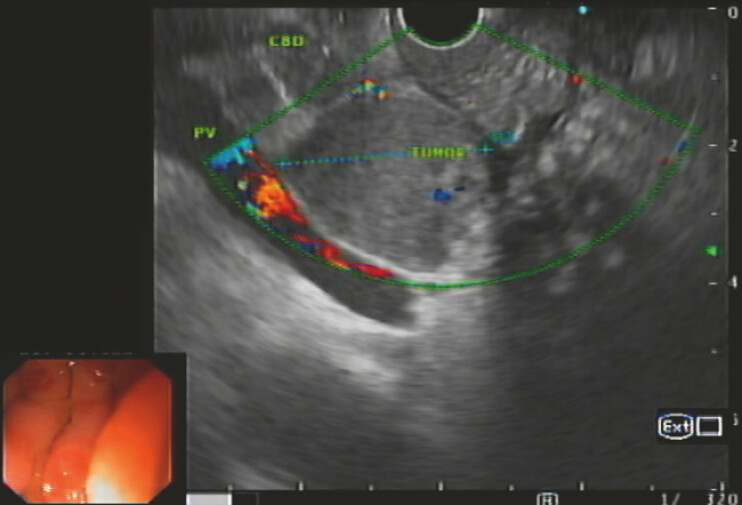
An endoscopic ultrasound image showing common bile duct (CBD) dilation caused by the pancreatic head lesion.
